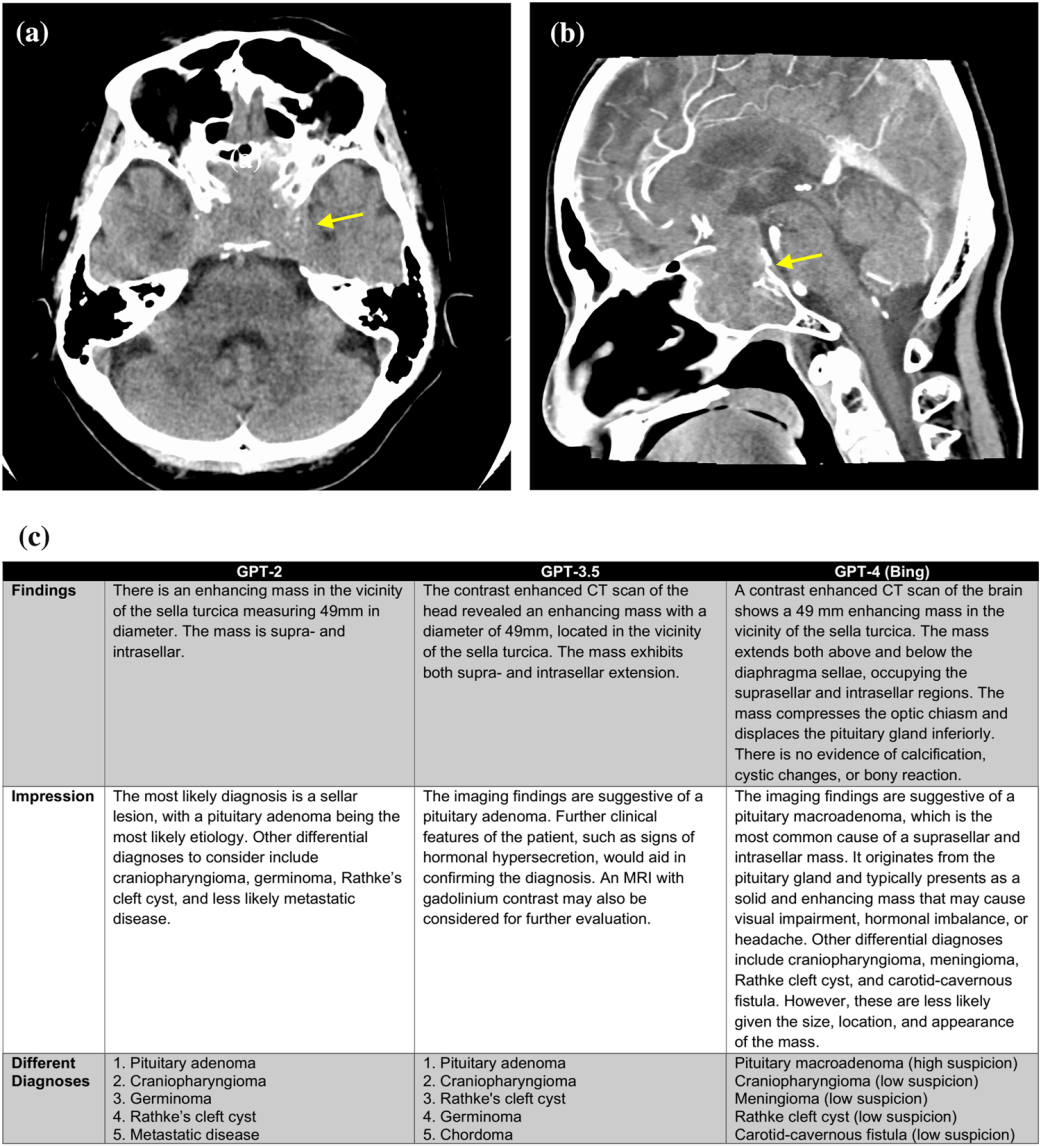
A 69-year-old female patient with a suspected pituitary adenoma. Non-contrast CT axial image (a), contrast-enhanced CT sagittal image (b) and generated radiology reports by GPT series (c) are shown. A tumor with homogeneous enhancement is observed from the sella turcica to the suprasellar region, suggesting a pituitary adenoma. Information input other than the prompt is “Age (years): 69, sex: female, modality: contrast enhanced CT, location: the vicinity of the sella turcica, diameter: 49 mm, findings: enhancing supra- and intrasellar mass”. The GPT-2 report is a simple report written according to the input information, and the differential diagnosis seems relatively reasonable. In the GPT-3.5 report, both the findings and impression sections are more detailed than in the GPT-2 report. The GPT-4.0 report is overall quite similar to a human-generated report, and the differential diagnosis is reasonable. However, it includes information that was not input, such as calcification and cystic degeneration
